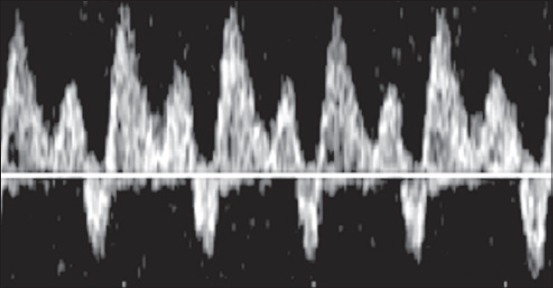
Pulsed-wave Doppler image of a triphasic waveform of a ductus venosus.
